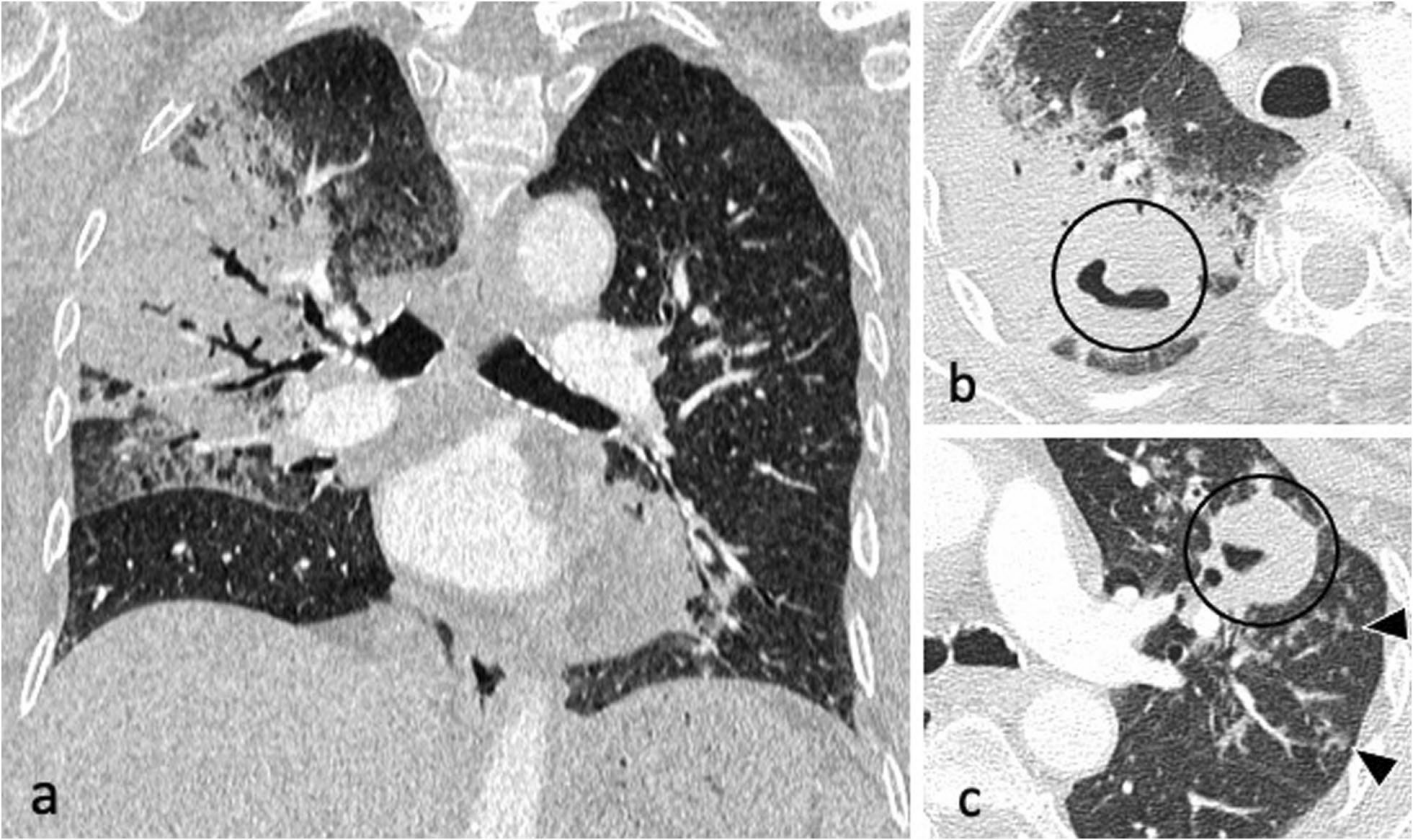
An 83-year-old male presented with pyrexia of unknown origin. CT (lung window) demonstrates extensive consolidation in the right upper lobe (coronal plane—a), bilateral thick-walled cavities (axial planes—b, c, circled) and centrilobular nodules with a tree-in-bud appearance (arrowheads)
